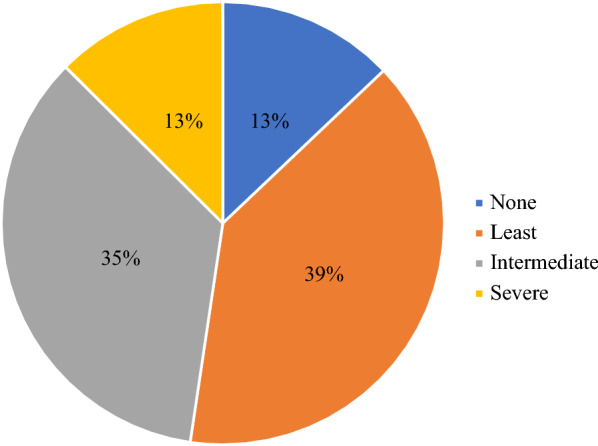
Diabetic cardiomyopathy subtypes at baseline (n = 804). None = no abnormal echocardiographic parameter. Least restrictive = 1 abnormal echocardiographic parameter (diastolic impairment, dilated left atrium or increase LV mass). Intermediate restrictive = at least 2 abnormal echocardiographic parameters and NTpBNP level within the normal range (< 125 pg/mL). Severe = at least 2 abnormal echocardiographic parameters and an elevated NTpBNP level (≥ 125 pg/mL)
